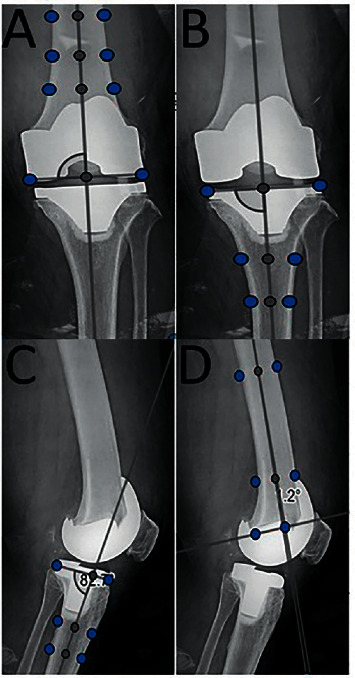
Radiographic outcomes. (a) Alpha (*α*) angle; (b) beta (*β*) angle; (c) delta (*δ*) angle; (d) gamma (*γ*) angle.
